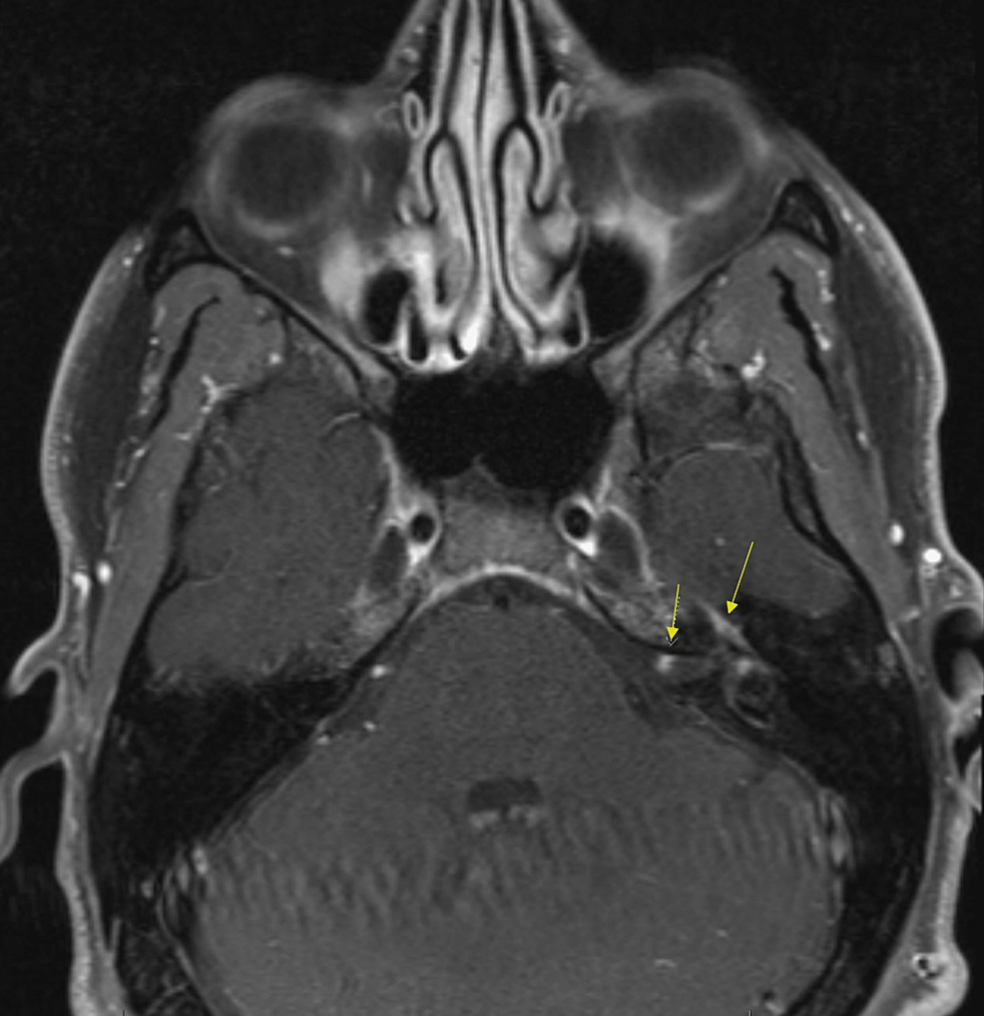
Axial T1 fat-saturated sequence post-contrast MRI of the brain seven months after initial imaging The image shows decreased enhancement of the left internal auditory canal (IAC) and stable asymmetric enhancement of the left geniculate ganglion, tympanic segment, and descending mastoid segment of cranial nerve seven, apical turn of the cochlea, vestibule, and lateral and posterior semicircular canal (arrows).
